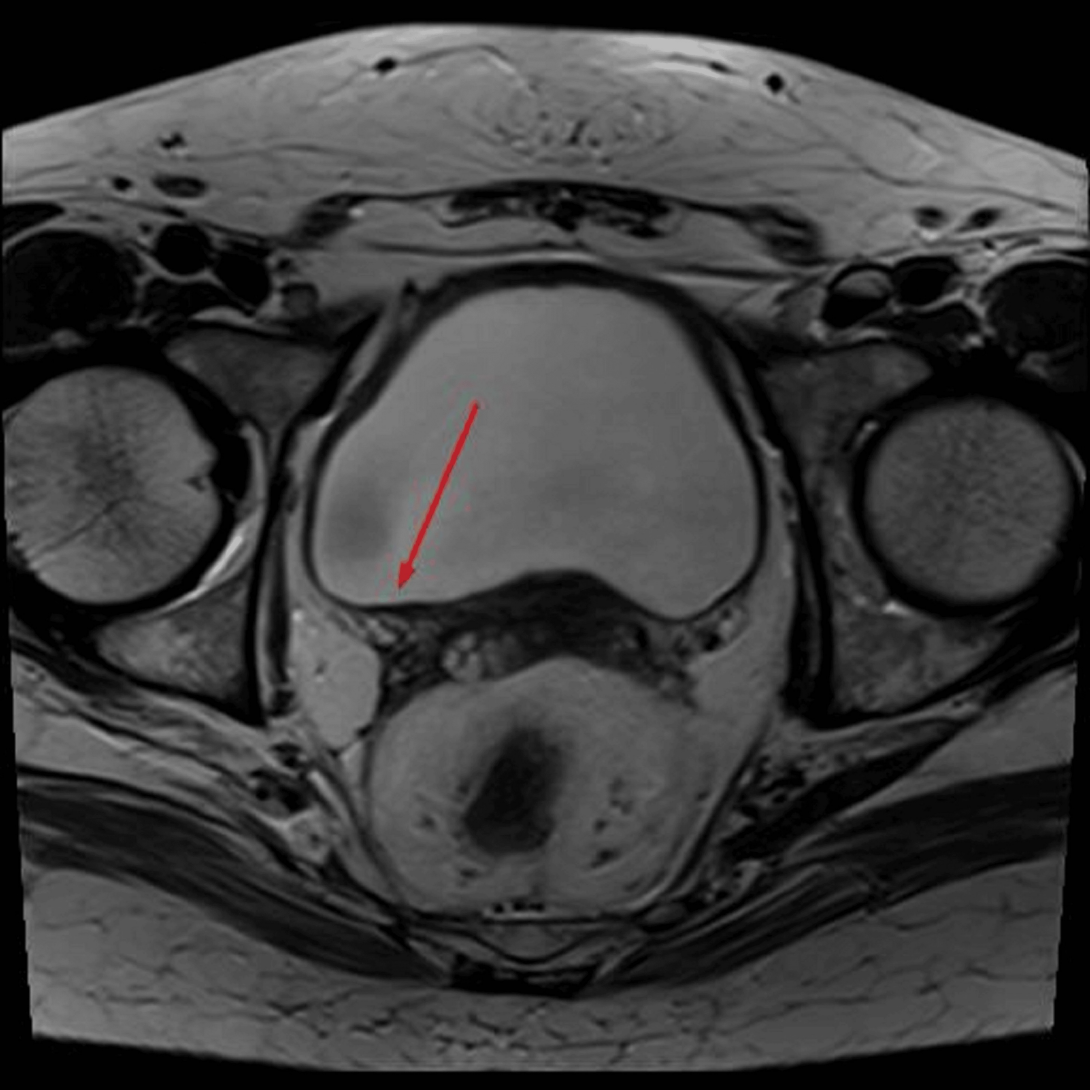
Resolved bladder wall lesion can be seen in MRI T2 images.
